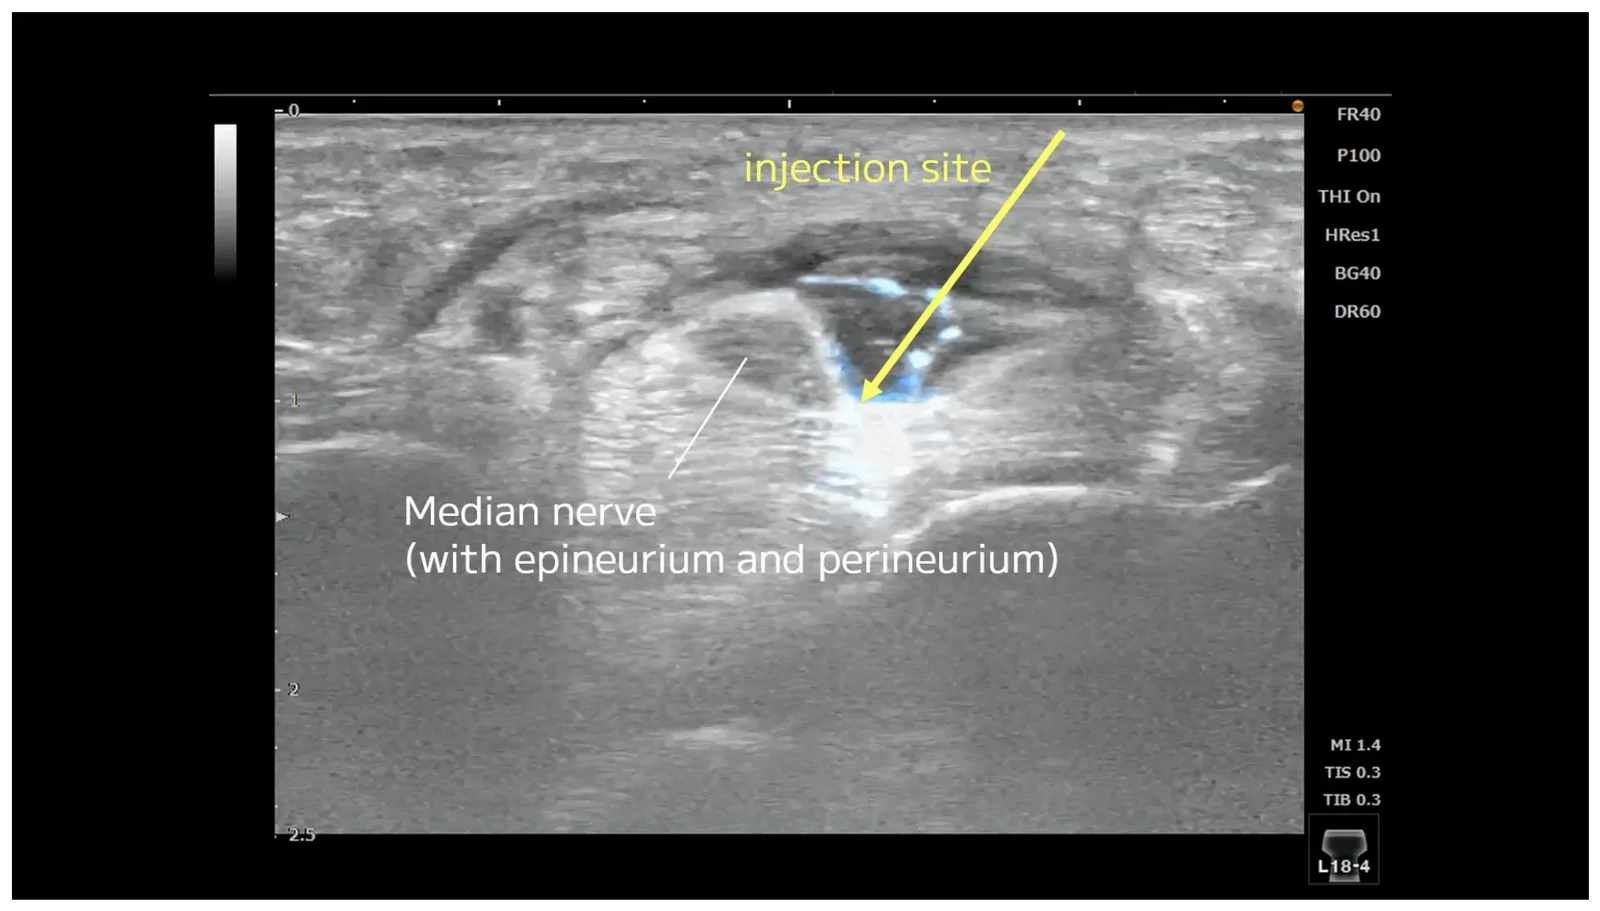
Point 12—median nerve–paraneural sheath/interfascicular epineurium: ultrasound-guided fascia hydrorelease; yellow arrow indicates the injection site.
